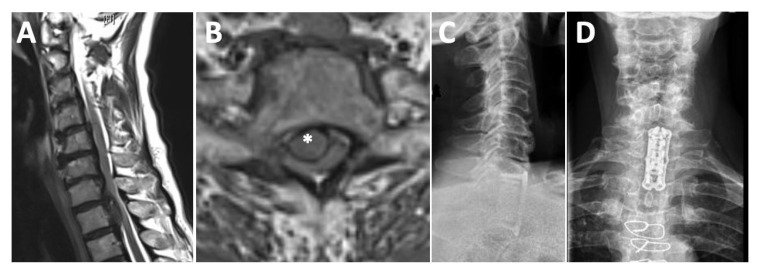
Junctional disc herniation. Preoperative T2-weighted MRI in sagittal (A) and axial (B) orientation showing a large C7/T1 central disc herniation with caudal extension to T2, severe spinal cord compression, and acute myelopathy (herniated disk is highlighted by * in (B)). Postoperative X-ray in lateral (C) and anterior-posterior (D) view, confirming excellent implant position after standalone anterior treatment.
